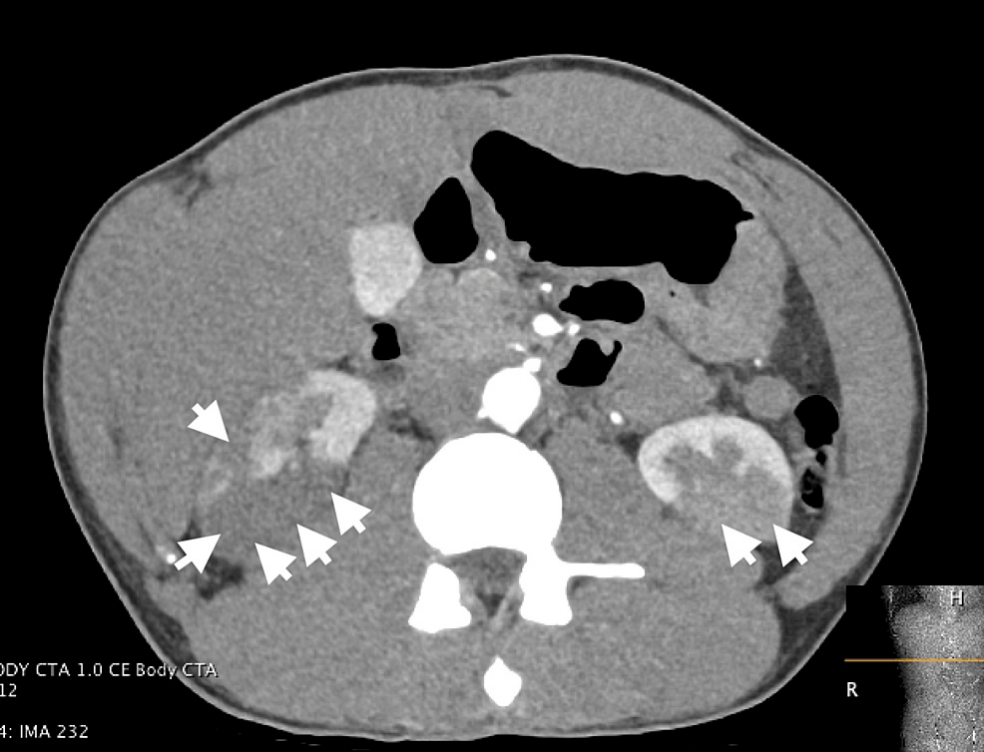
CT abdomen showing bilateral renal infarcts and high-grade stenosis of the inferior segmental branch of the right renal artery and narrowing of inferior segmental branches.
